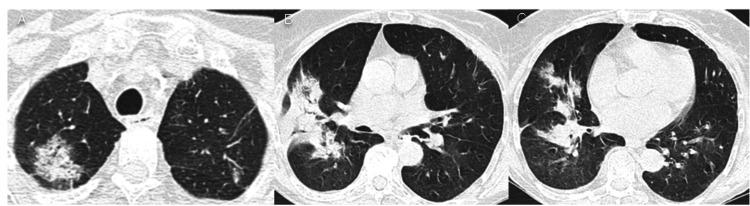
Chest CT five months after hospital discharge. Migratory pulmonary consolidations.
